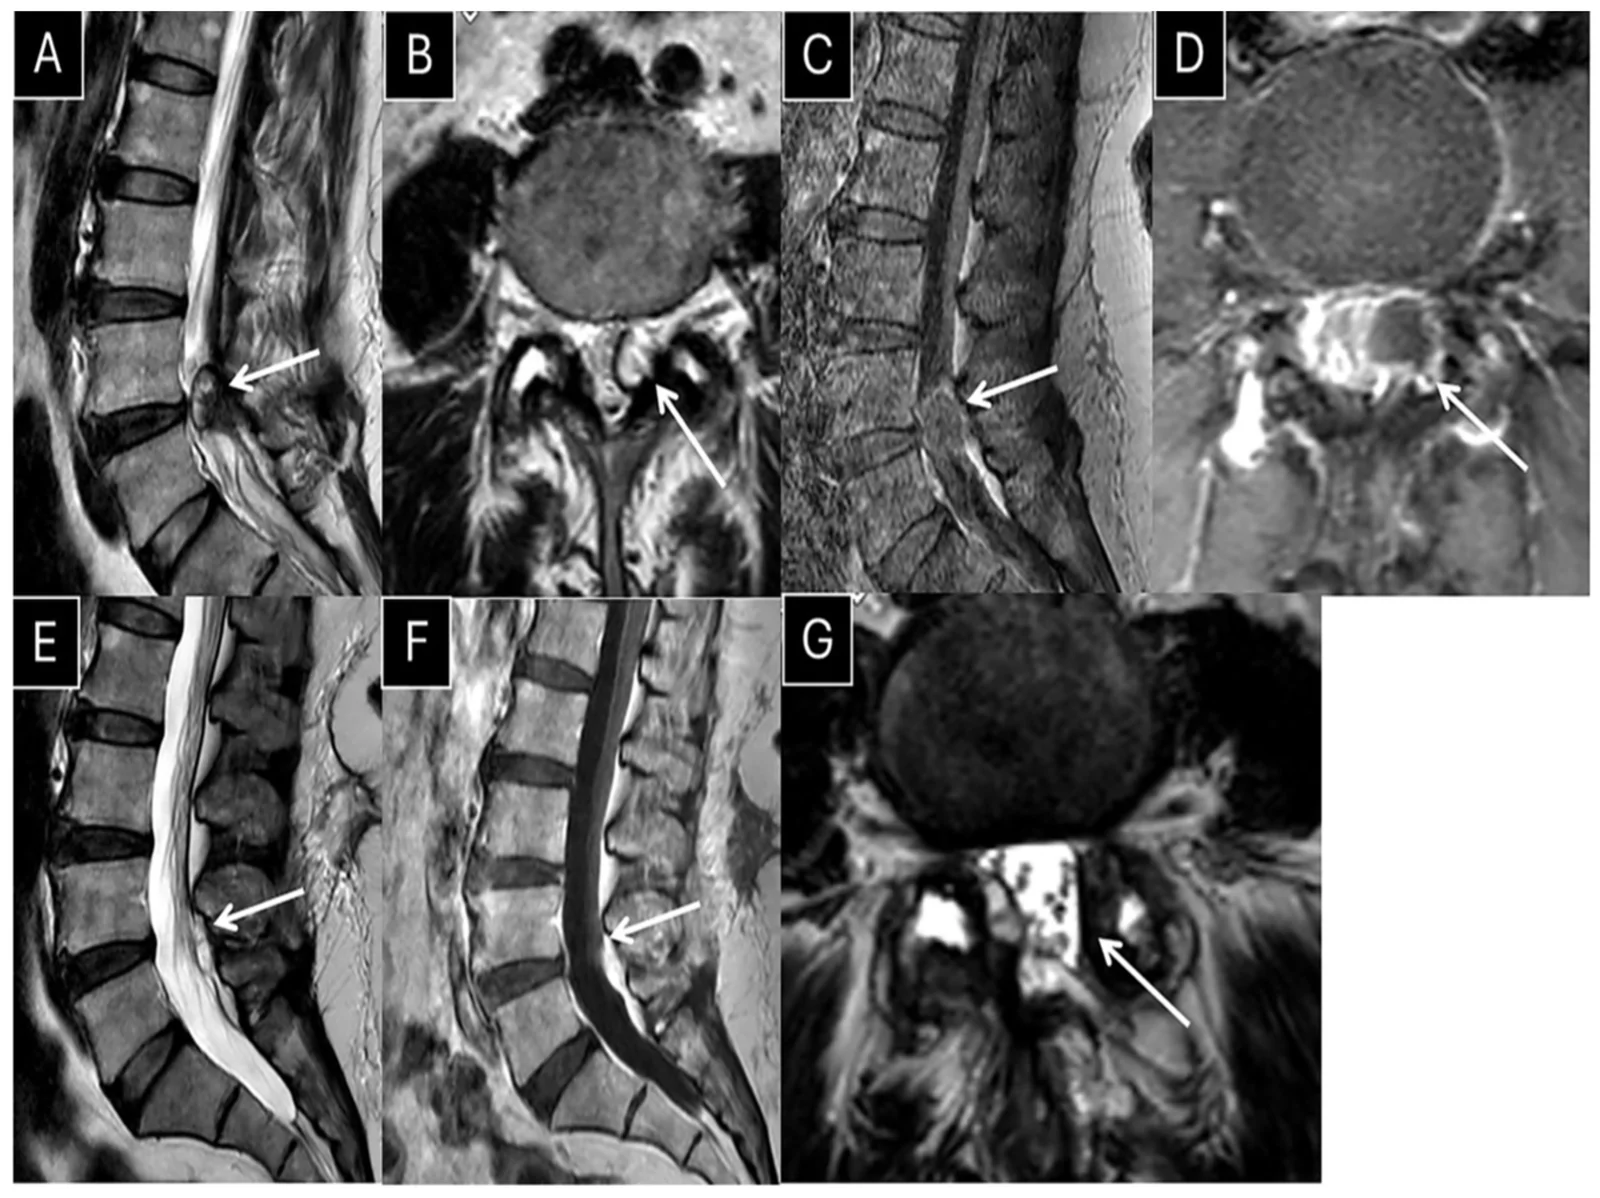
Representative case of lumbar synovial cyst at the L4–L5 level. (A) Preoperative sagittal T2-weighted MRI demonstrating a lumbar synovial cyst causing marked compression of the thecal sac (arrow). (B) Preoperative axial T2-weighted MRI demonstrating spinal canal compression by the lumbar synovial cyst (arrow). (C) Preoperative sagittal contrast-enhanced T1-weighted MRI demonstrating the lumbar synovial cyst (arrow). (D) Preoperative axial contrast-enhanced T1-weighted MRI demonstrating the lumbar synovial cyst adjacent to the facet joint (arrow). (E) Postoperative sagittal T2-weighted MRI demonstrating complete excision of the cyst and satisfactory decompression of the thecal sac (arrow). (F) Postoperative sagittal contrast-enhanced T1-weighted MRI demonstrating no residual lumbar synovial cyst (arrow). (G) Postoperative axial T2-weighted MRI demonstrating adequate spinal canal decompression following cyst excision (arrow).
